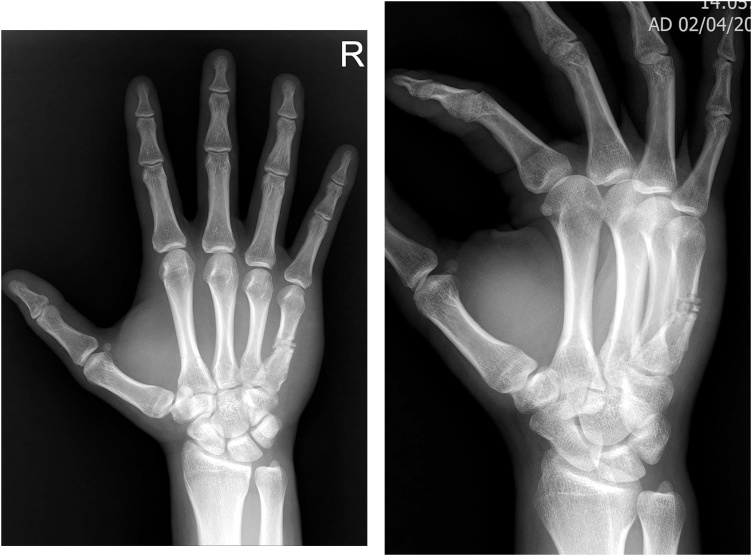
Simple X-ray (AP view (left) and oblique view (right) Postoperative 8 weeks. Initial CMC dislocation and fracture was well healed, however displacement of fracture on metacarpal body caused by secondary fracture is aggravated and malunion was suspected.
